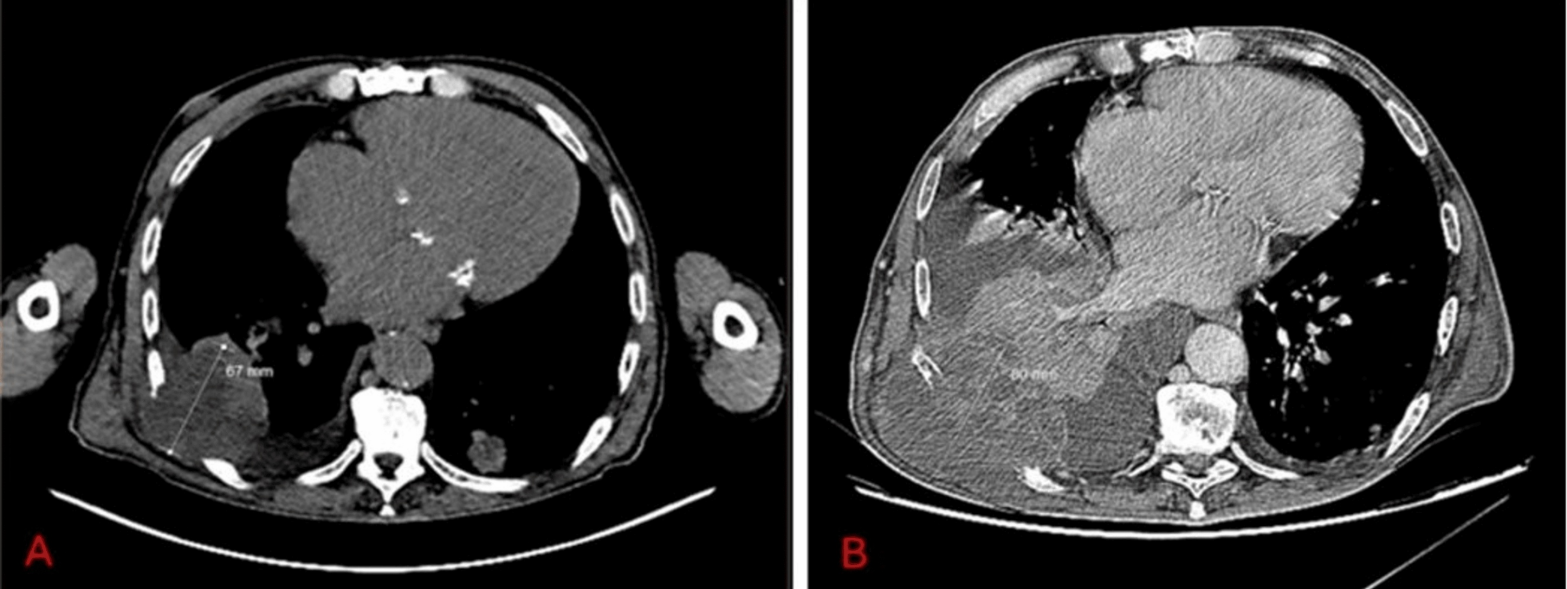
Comparative images from computed tomography scans performed at treatment baseline (A) and three months after treatment discontinuation (B), evidencing disease progression according to the RECIST criteria A: target lesion measuring 67 mm; B: target lesion measuring 80 mm + new lesions on lymph nodes and a new onset pleural effusion.
